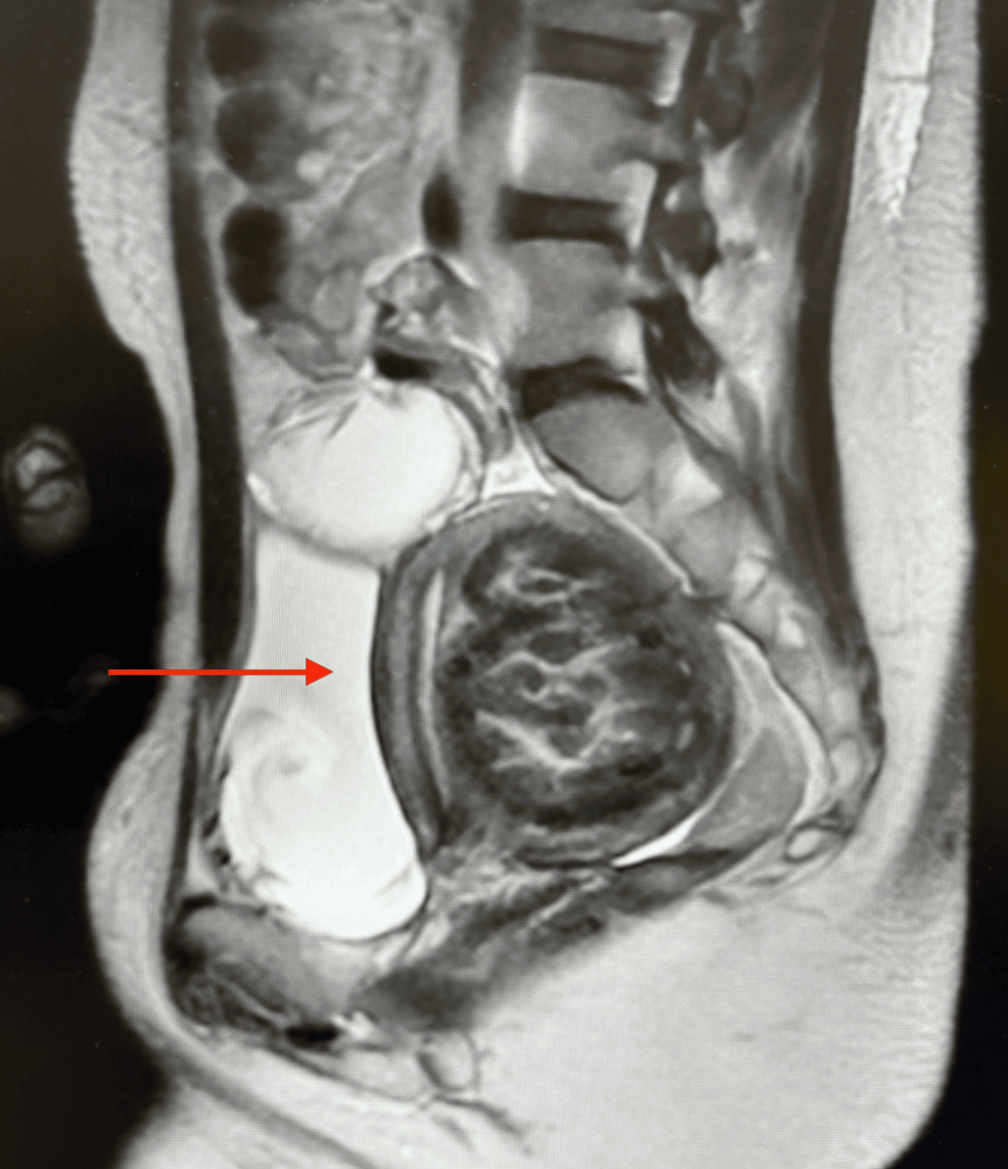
MRI of the pelvis displaying enlarged fibroid uterus with a 7 cm mass causing endometrial displacement.
